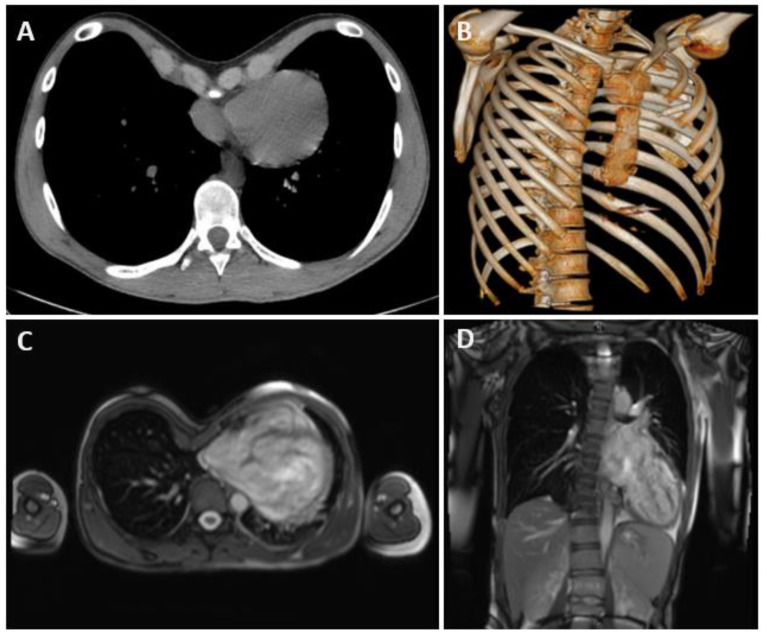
(A,B) Chest computed tomography and 3D reconstruction showing severe pectus excavatum (PE) in patient with Micra leadless pacemaker (MLP). (C,D) Cardiac magnetic resonance in a Marfan patient with severe PE and heart displaced to the left.
